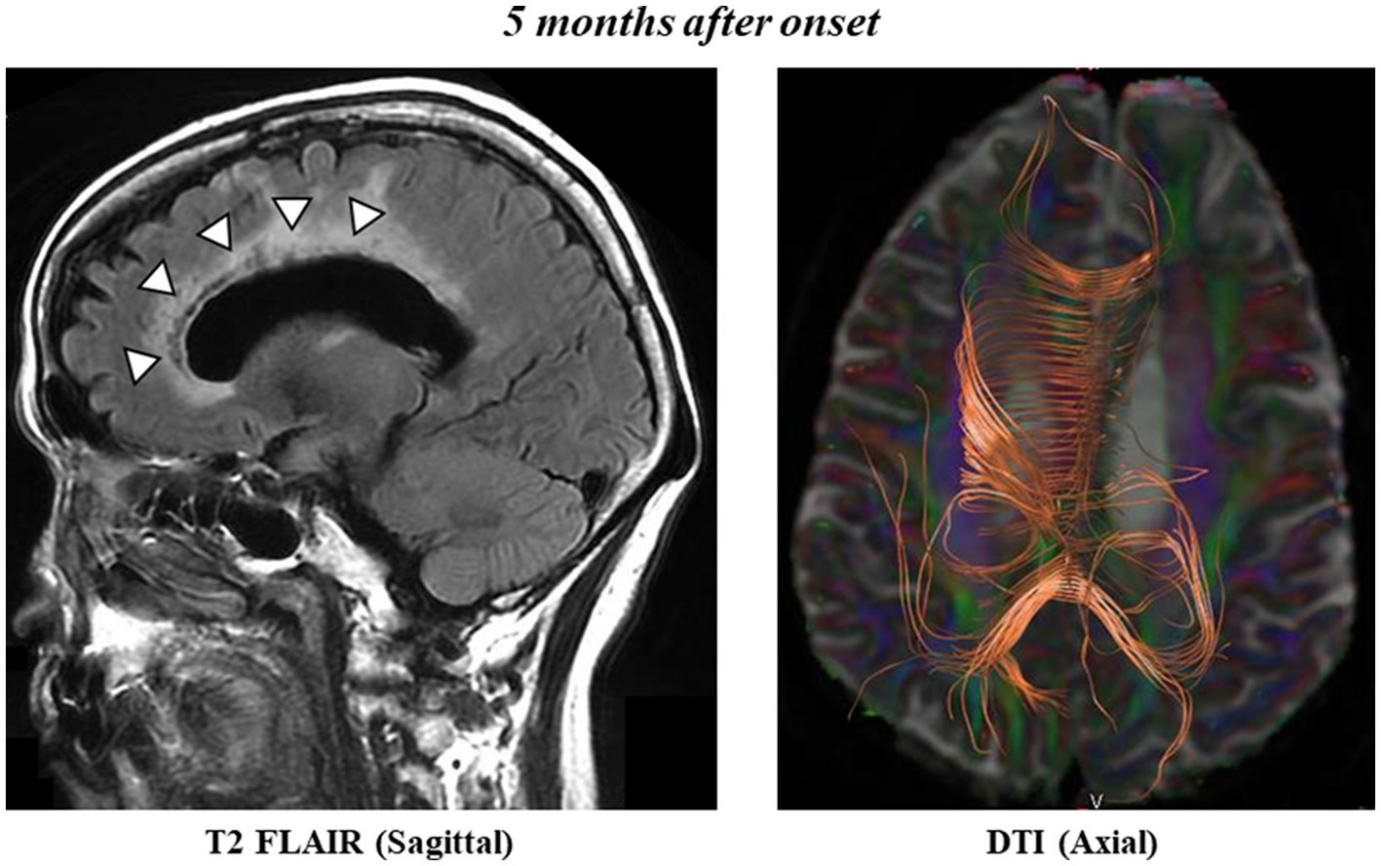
Sagittal T2 FLAIR scan shows postinfarct necrosis in the left frontal lobe, genu, and midbody of the CC 5 months after onset (left panel). Axial diffusion tensor tractography of the CC 5 months after onset (right panel).
